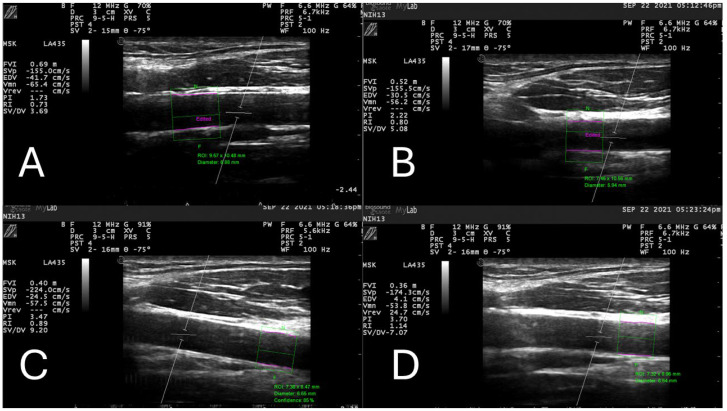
Representative ultrasound images and common carotid artery (CCA) diameter measurements of one subject during resting (A) and low-intensity (B), moderate-intensity (C), and high-intensity (D) exercise conditions. In all 4 images, the green dotted squares represent the region of interest (ROI), where the edge detection software (Vascular Research Tools, Medical Imaging Applications LLC, Coralville, IA, USA) identifies the edges of the CCA represented by the purple lines and measures the vessel’s diameter (values written in green letters).
